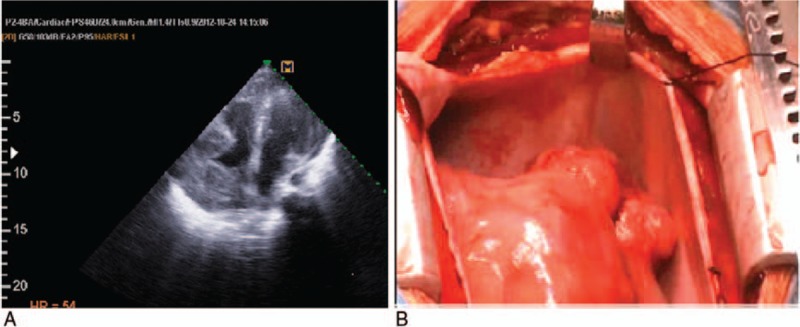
(A) Transthoracic echocardiography, 2 echogenic masses with lucent centers on RA free wall and RVOT. (B) Two large extra cardiac masses with pressure effect on RVOT.
